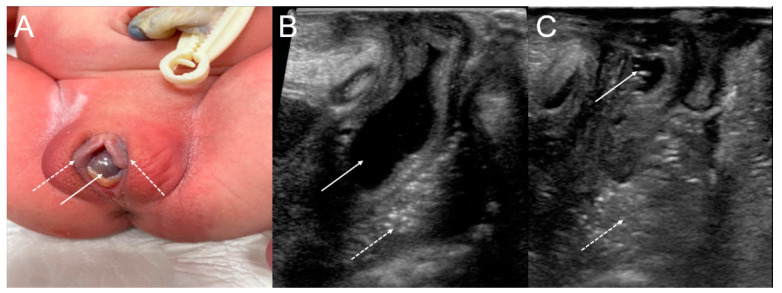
(A) The cystic bulging mass (arrow) arising from the right vaginal wall between the labia minora (dotted arrow) after birth. (B) On the initial ultrasound with a perineal approach, a fluid-filled cystic lesion (arrow) in the right pelvic cavity was noted anterior to the rectum (dotted arrow). (C) After aspiration, the previously observed cystic lesion collapsed (arrow).
